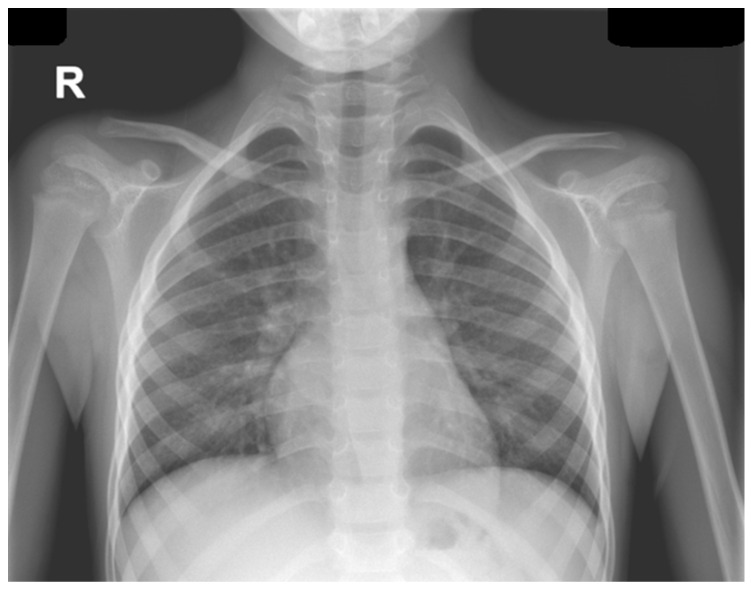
Anteroposterior cardiothoracic radiography, Case 2.
